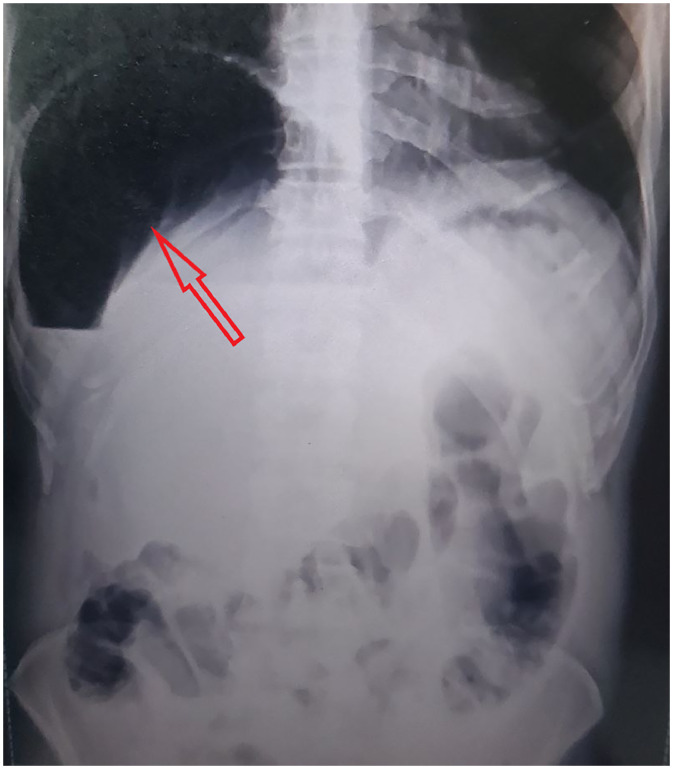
Erect anteroposterior abdominal radiograph demonstrating the interposition of a gas-distended colonic segment between the liver and the right hemidiaphragm, characterized by the presence of visible haustral markings within the subphrenic lucency, and significant gaseous dilatation of the proximal colonic loops is noted.
